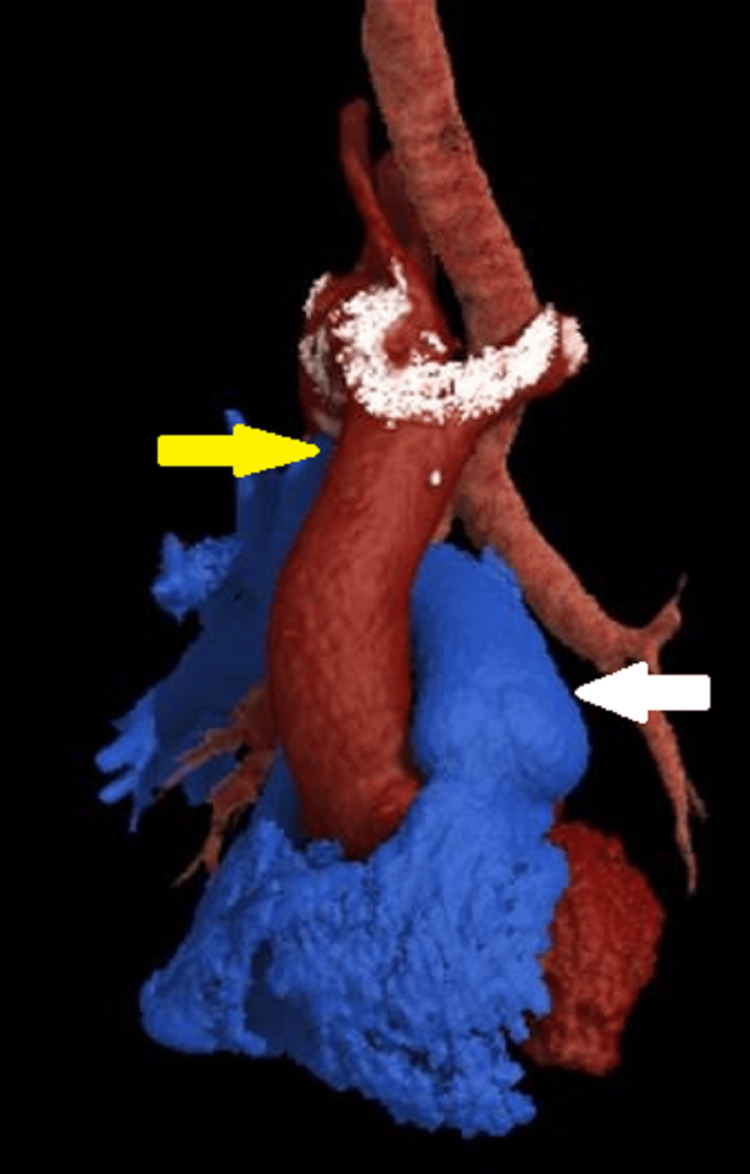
Volume-rendered three-dimensional (3D) CT angiographic image. The image depicts the vascular abnormalities, including unilateral absence of the left pulmonary artery (white arrow), a right-sided aortic arch, and its relationship with the trachea (yellow arrow).
